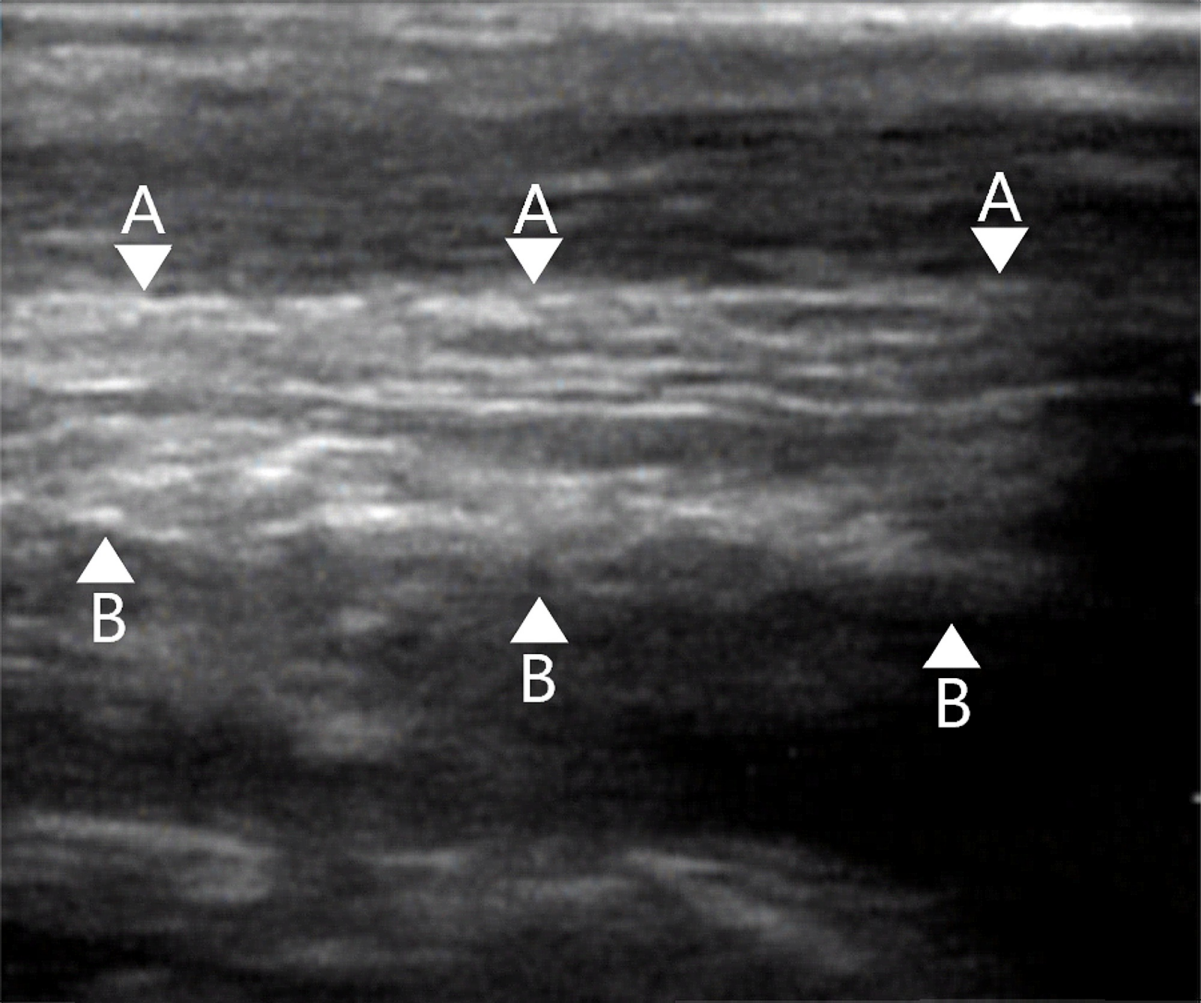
Ultrasound of a normal Achilles tendon in the longitudinal axis showing a continuous linear pattern which is fibrillar and hyperoechoic. Ultrasound images from Kopinksi and Davis, 2020 [14] A- Intact anterior aspect of Achilles tendon B- Intact posterior aspect of Achilles tendon Original images authorised by respective owners
